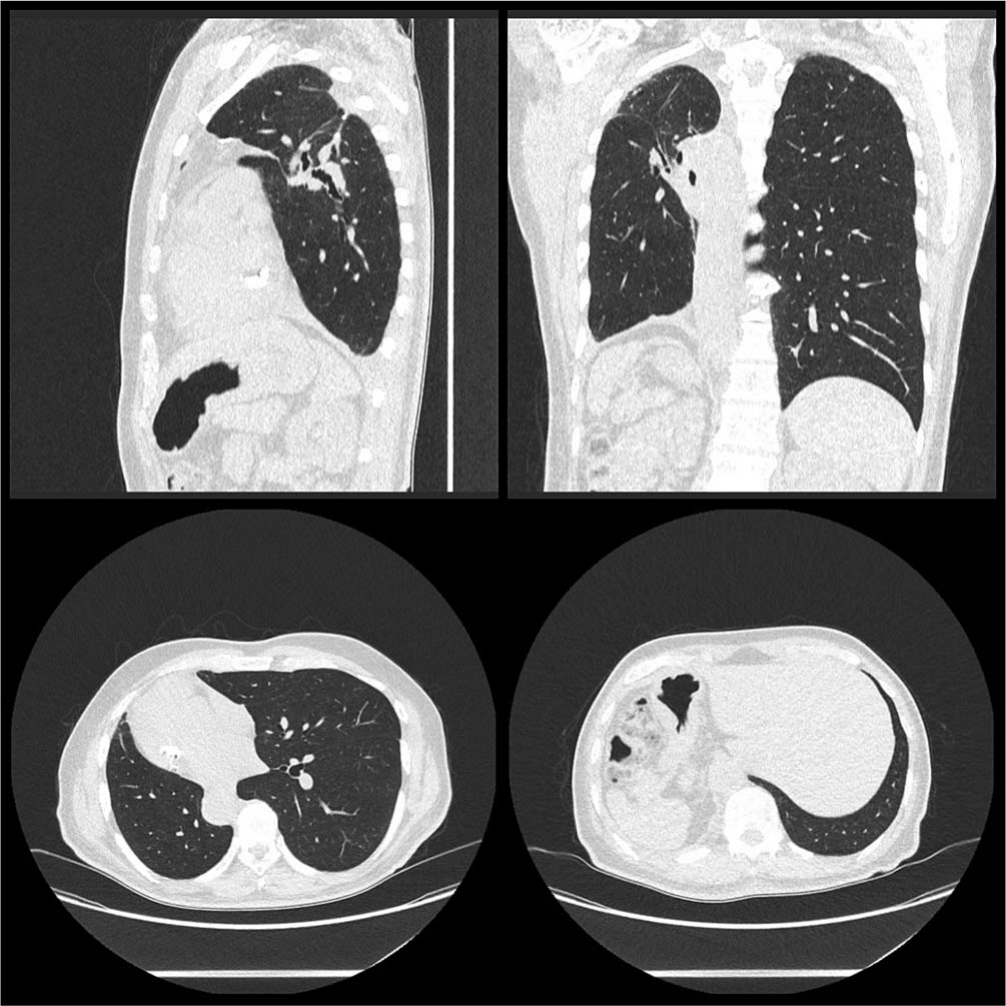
Post-operative CT thorax, abdomen, pelvis.
